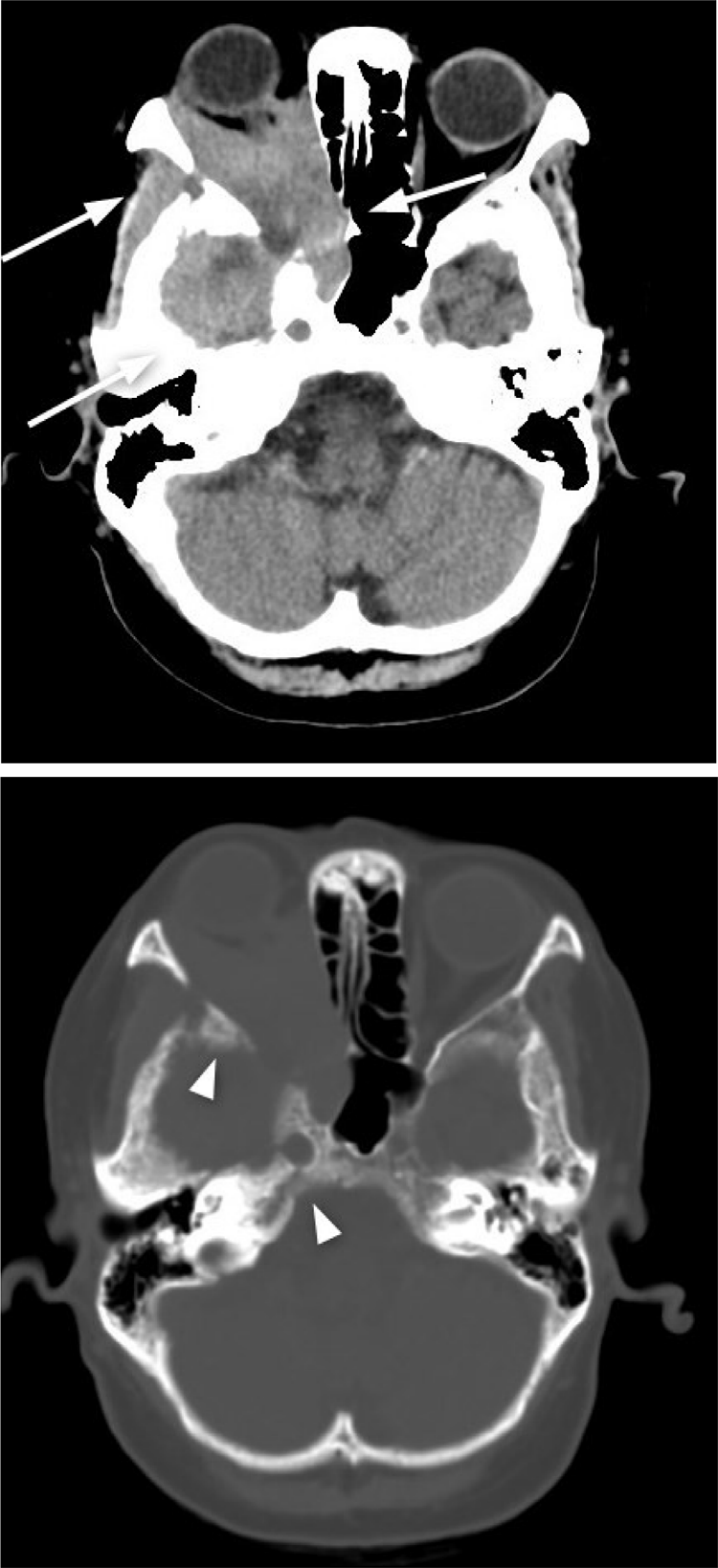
CT image demonstrates a mildly high-density lesion (arrows, A) occupying most of the right orbit extending to sphenoid sinus, skull base, and middle cranial fossa with bone erosion (arrowheads, B). There was no evident calcification.
